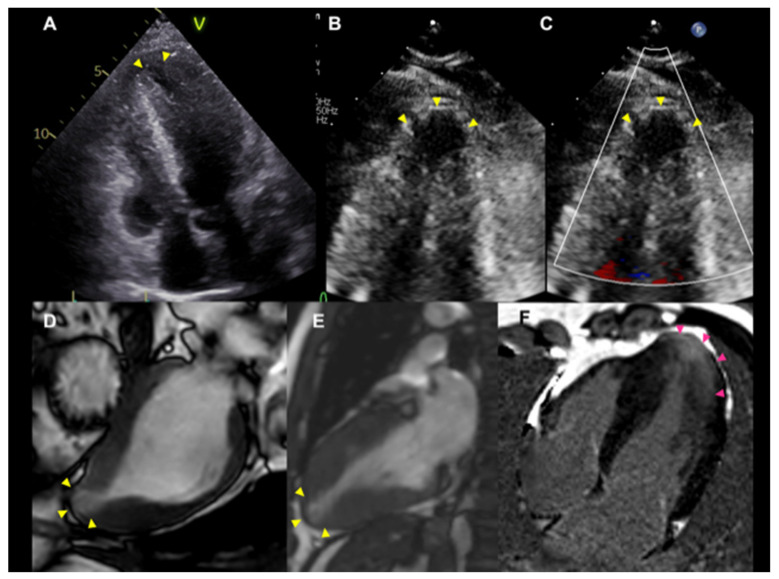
Representative cases with left ventricular apical aneurysm. Transthoracic echocardiography (A–C) and cardiac magnetic resonance imaging (D–F). Yellow arrowheads indicate apical aneurysms and red arrowheads indicate late gadolinium enhancement.
